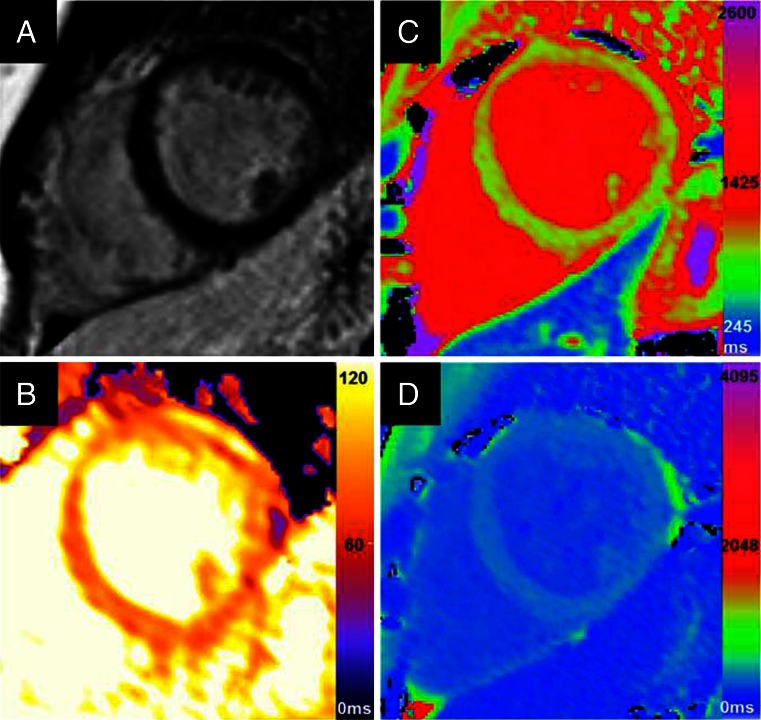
Cardiac magnetic resonance images from a 48-year-old patient with systemic sclerosis (SSc), with a normal left ventricular (LV) ejection fraction of 63 %. a LGE imaging with no focal areas of enhancement. b T2 map. c Native T1 map (acquired using ShMOLLI at 1.5 T) showed significantly elevated average LV myocardial T1 values of 1009 ± 47 ms (normal 962 ± 25 ms). d Post-contrast T1 map acquired at 15 min after gadolinium administration. Average post-contrast T1 values were 479 ± 18 ms. Extracellular volume (ECV) quantification using pre- and post-contrast T1 mapping and adjusting for the hematocrit demonstrated a significant expansion of interstitial space at 40 % (normal 27 ± 3 %). *Images courtesy Dr. Ntobeko Ntusi*, *University of Oxford*
